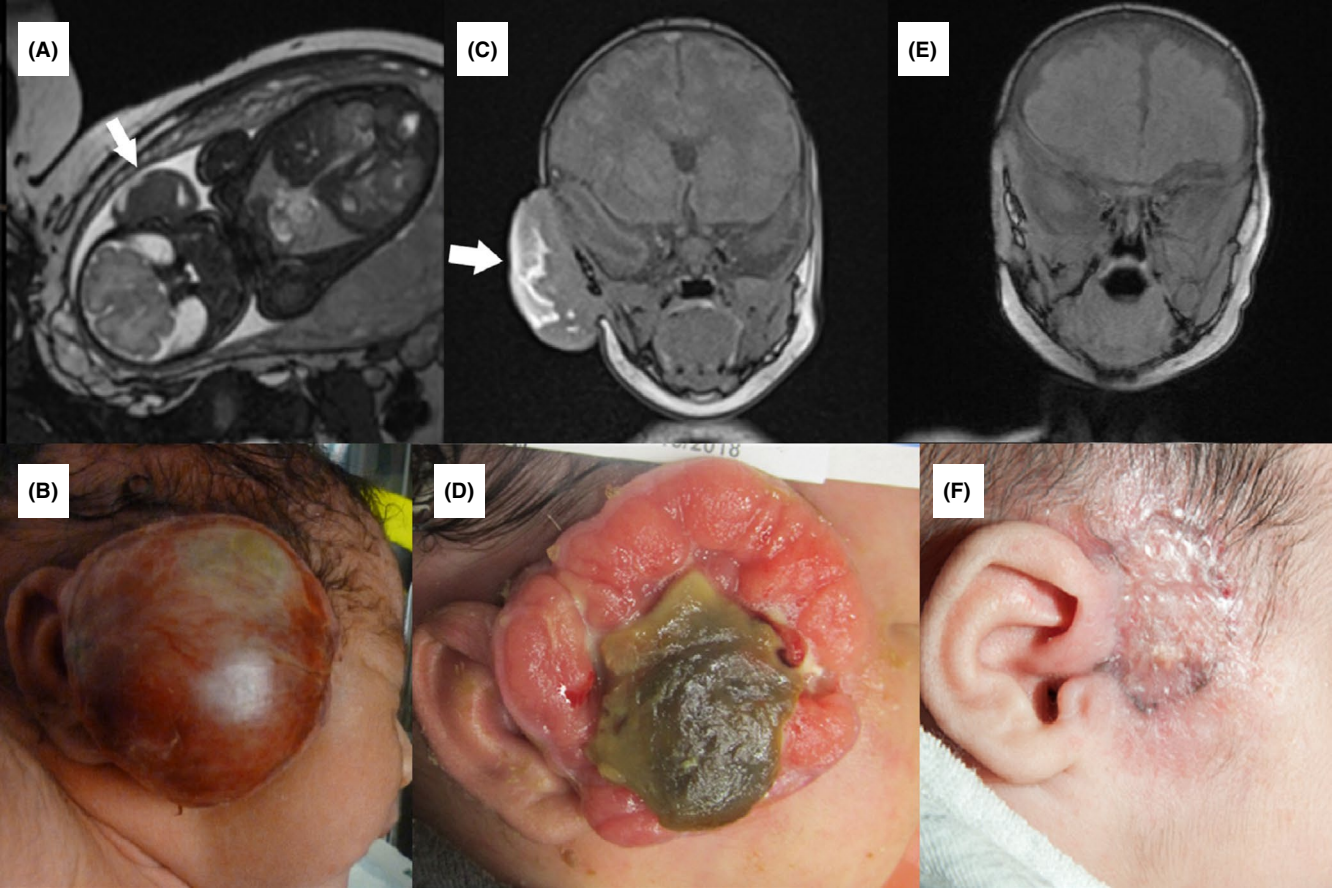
Myofibroma for Patient 2 on fetal MRI (A) and at birth (B). MRI and clinical appearance at one month of age, immediately prior to starting imatinib (C and D, respectively). MRI and clinical appearance after 2 months on imatinib monotherapy (E and F, respectively). Arrows designate facial myofibroma on imaging. Reprinted with permission from Wenger, et al^3^
